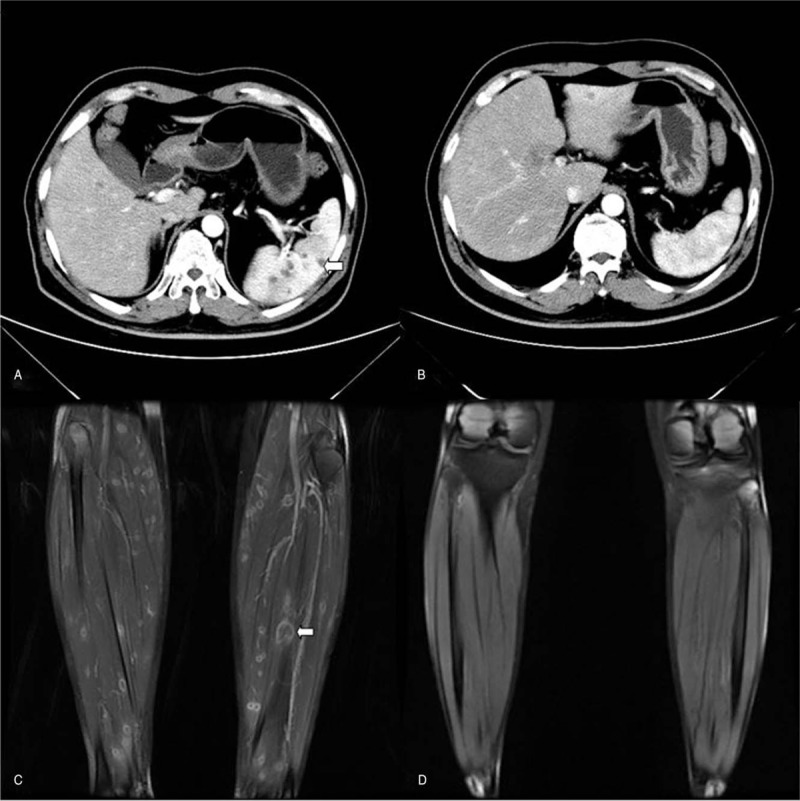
Imaging findings of spleen and legs before and after antifungal therapy (A) CT scan at initial showed a few hypodensities in the spleen; (B) Latest CT scan done at 2 months post antifungal treatment presenting improvement in the splenic lesions; (C) Magnetic resonance imaging scan after neutrophil recovery post chemotherapy showed worsening radiologic hypodensities within the calves; (D) Magnetic resonance imaging scan after antifungal treatment showed improving radiologic hypodensities within the calves. CT = computed tomography.
